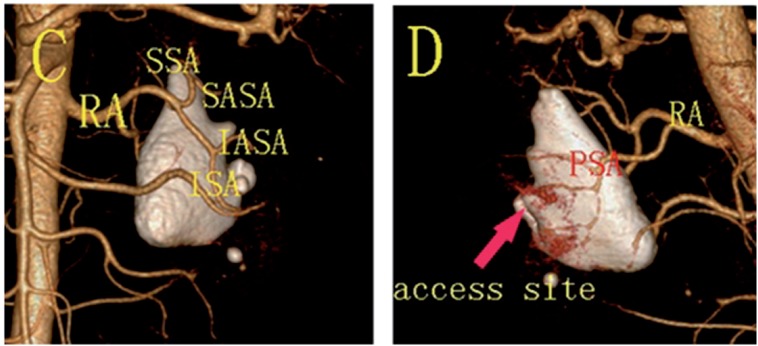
Computed tomography angiography.
